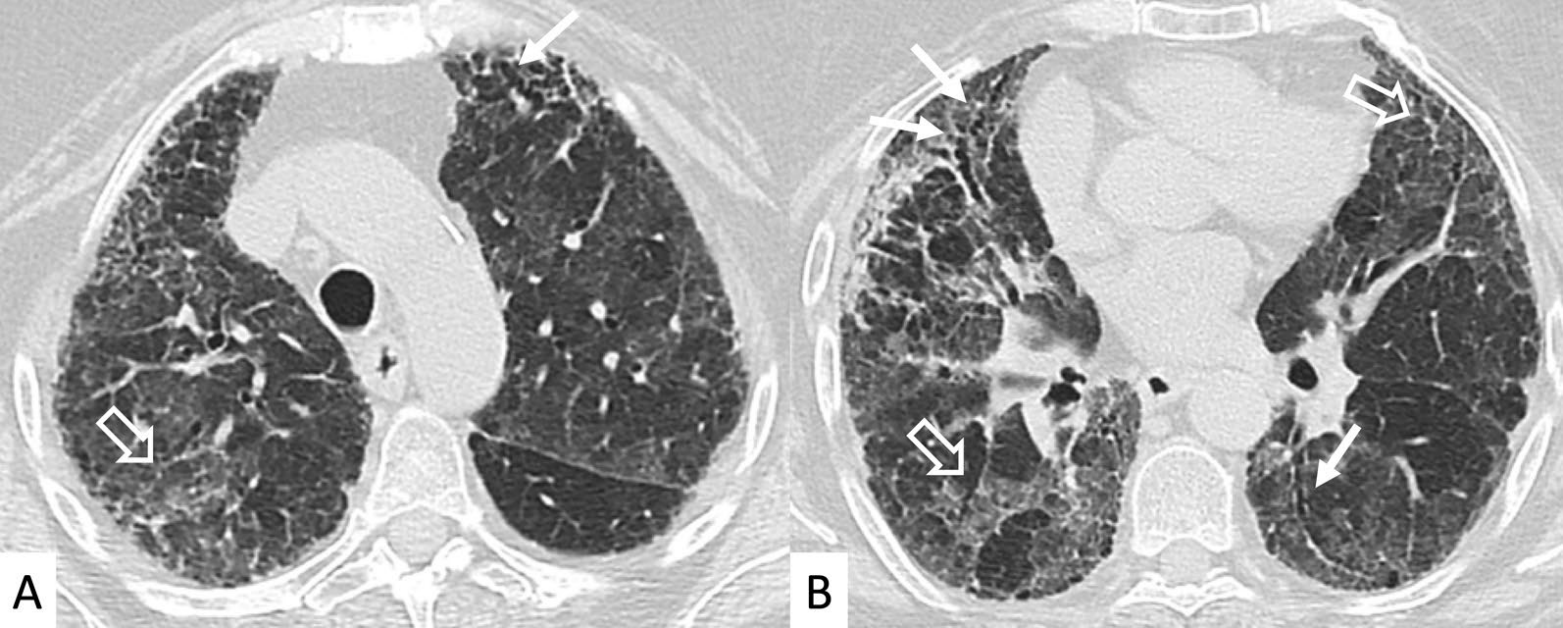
Axial HRCT images of a patient with rheumatoid arthritis. A, B clearly show ground-glass opacities (empty white arrows) and bronchiectasis (white arrows), resembling an NSIP pattern
